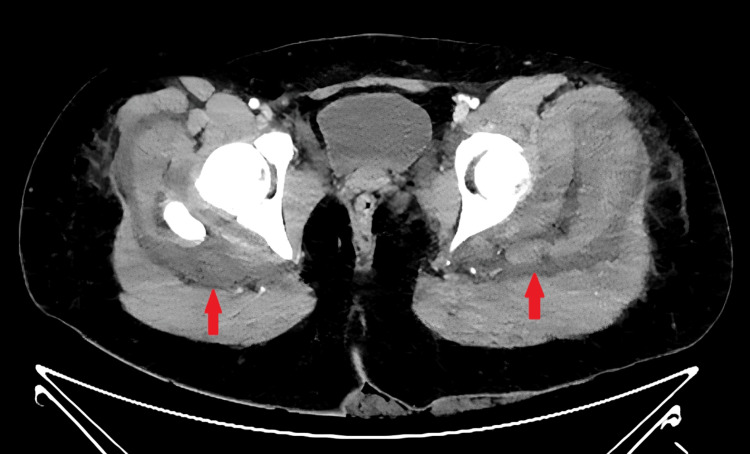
Axial post-contrast CT of the proximal lower extremity musculature Axial contrast-enhanced CT demonstrates bilateral gluteal muscle enlargement, muscle edema, and heterogeneous muscle enhancement. Arrows mark hypodense areas within the heterogeneous enhancement of the muscles.
